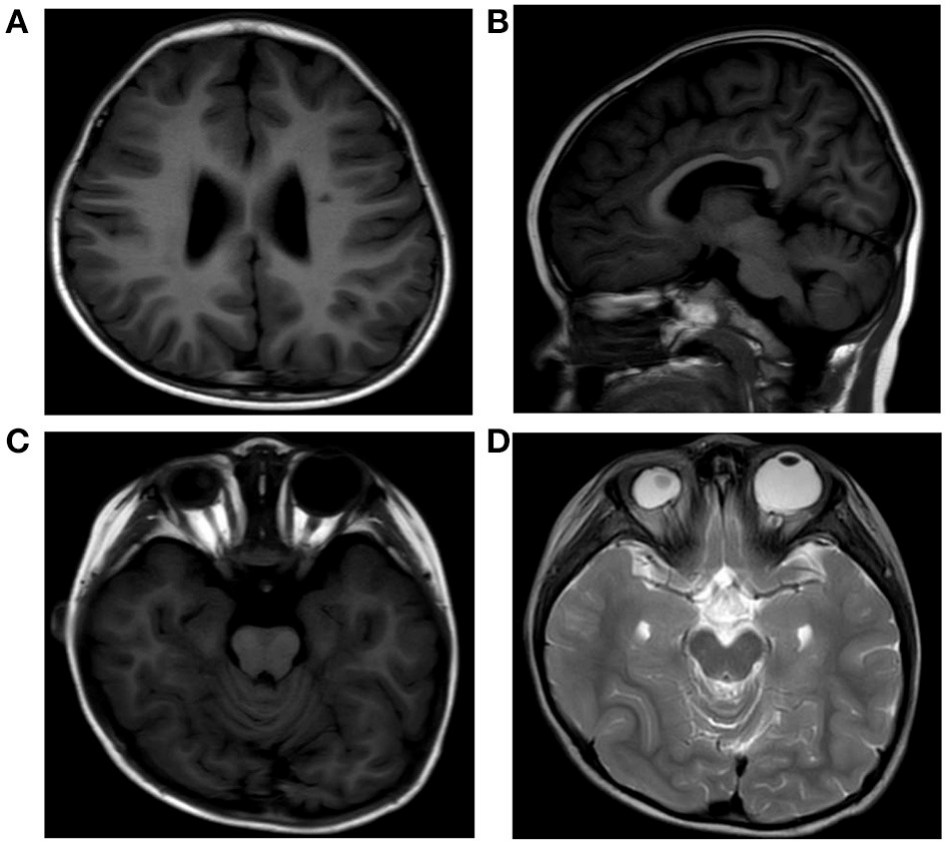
MRI of the patient's brain showing dilation of bilateral ventricles, a small patchy abnormal signal in paraventricular white matter (A), hypogenesis of corpus callosum (B), small right eye, and abnormal shape and signal of the lens (C,D).
